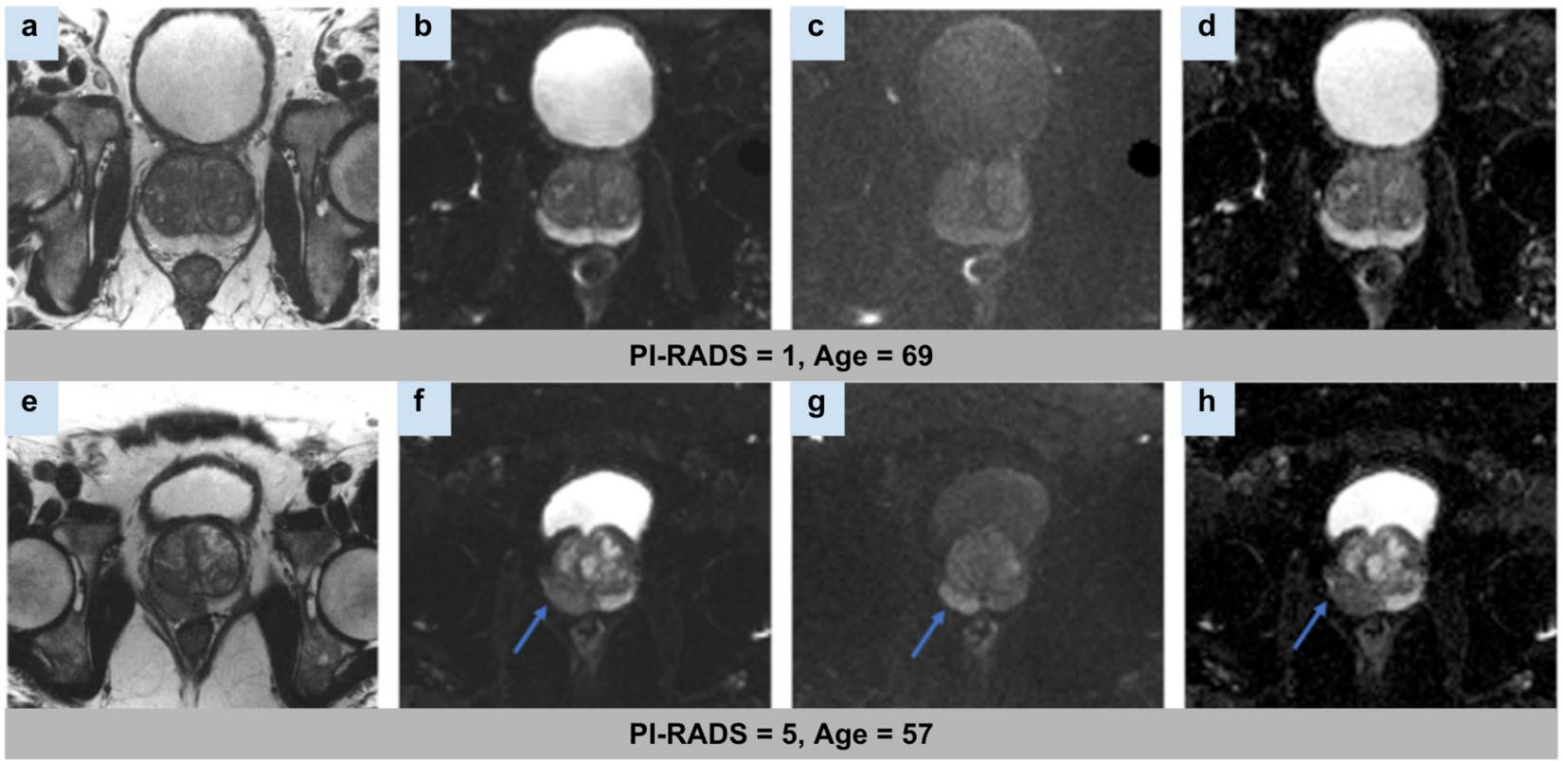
Example fastMRI prostate images. (Row 1) A reconstructed axial T2-weighted image (a), B50 DWI (b), B1000 DWI (c) and ADC Map (d) for a 69-year-old man referred for prostate evaluation, with a PI-RADS assessment of 1 based on the full set of acquired MR images. (Row 2) A reconstructed axial T2-image (e), B50 DWI (f) B1000 DWI (g) and ADC Map (h) for a 57-year-old man referred for clinical prostate MRI, with a PI-RADS assessment of 5. Blue arrows indicate a lesion in the right posteromedial midgland peripheral zone.
